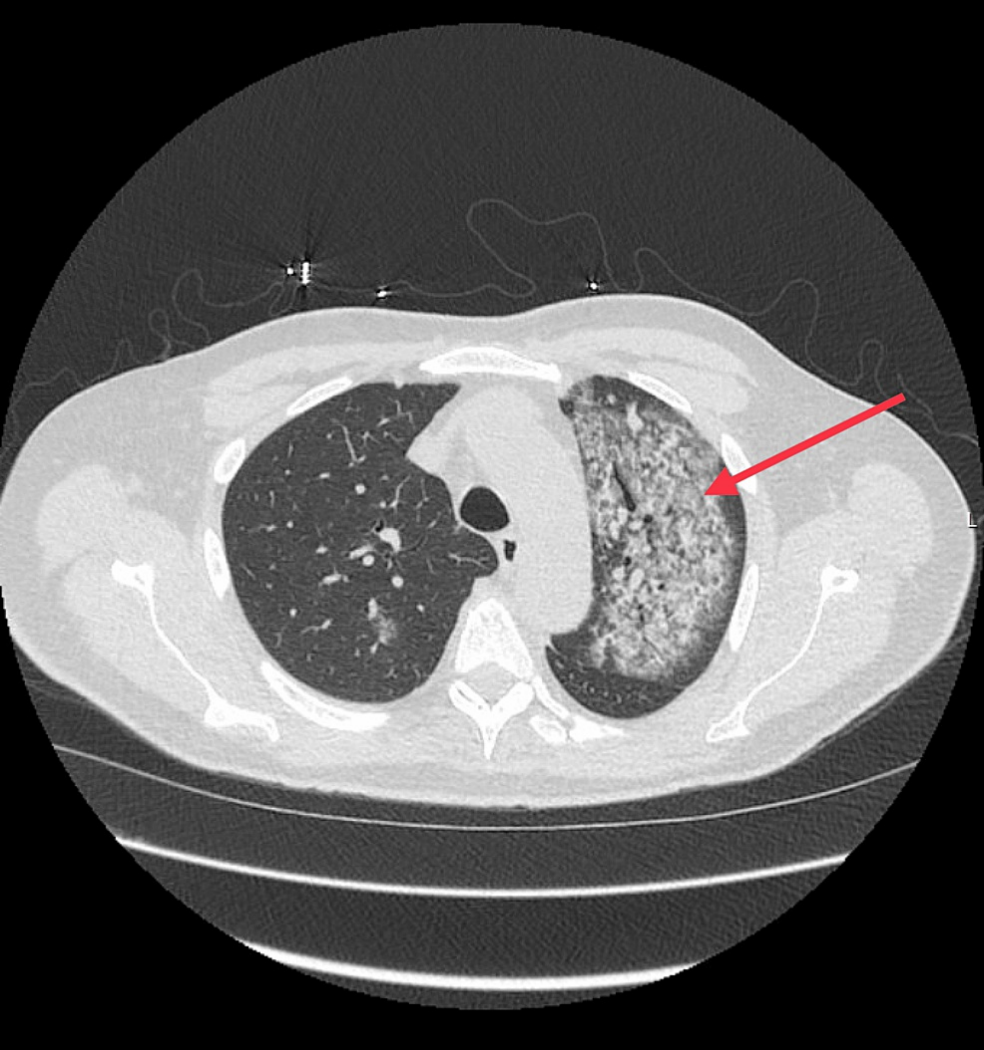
CT chest showing diffuse abnormal opacification (arrow)
